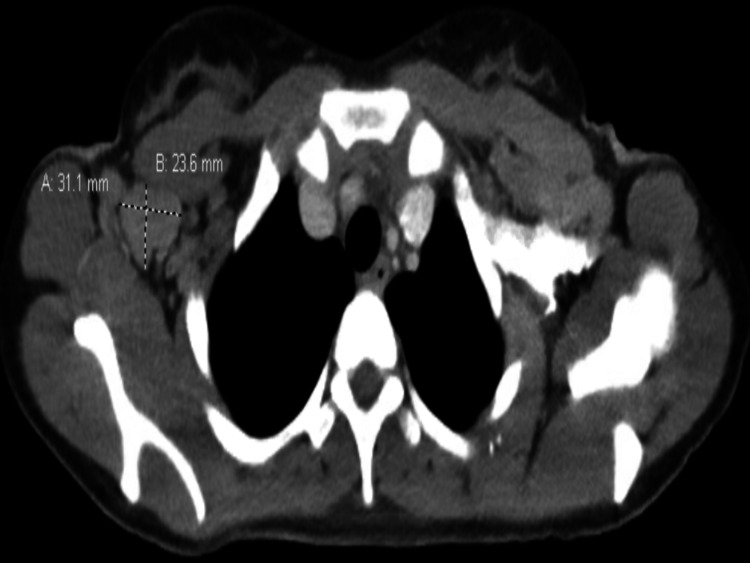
CT scan of chest with IV contrast showing bilateral axillary lymphadenopathy with the largest one measuring approximately 3.1 x 2.4 cm lymph node in the right axilla
